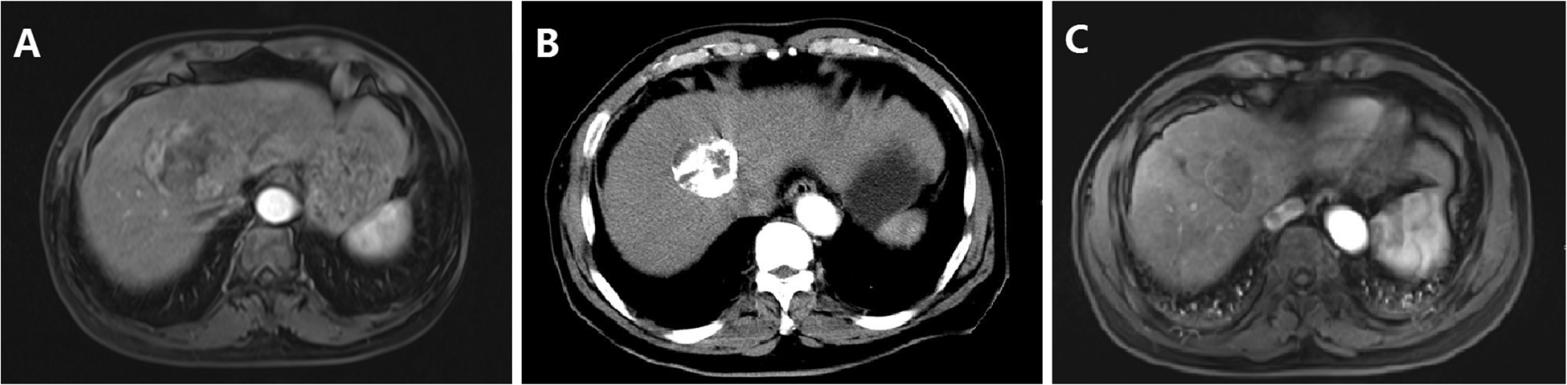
Complete ablation of HCC. A: MRI image before TACE; B: CT image before RFA; C: MRI image after RFA.
